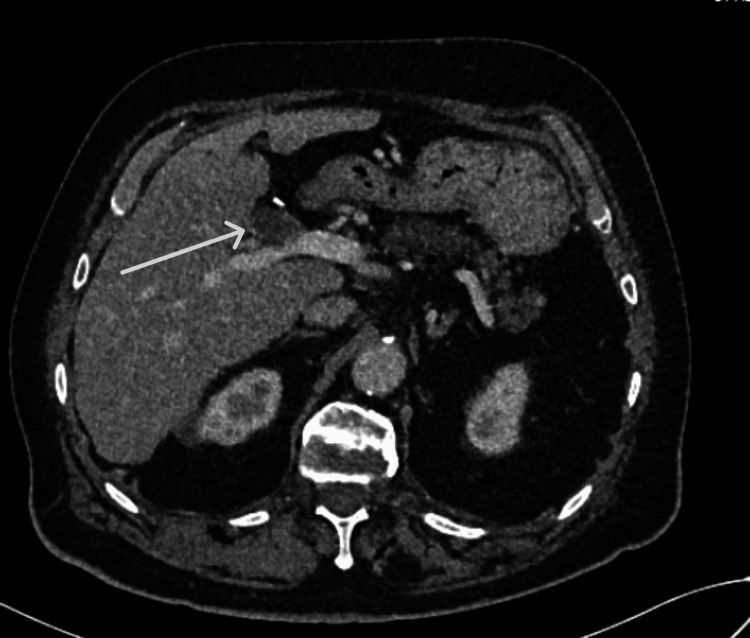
CT scan of the abdomen showing the initial left portal vein thrombosis
